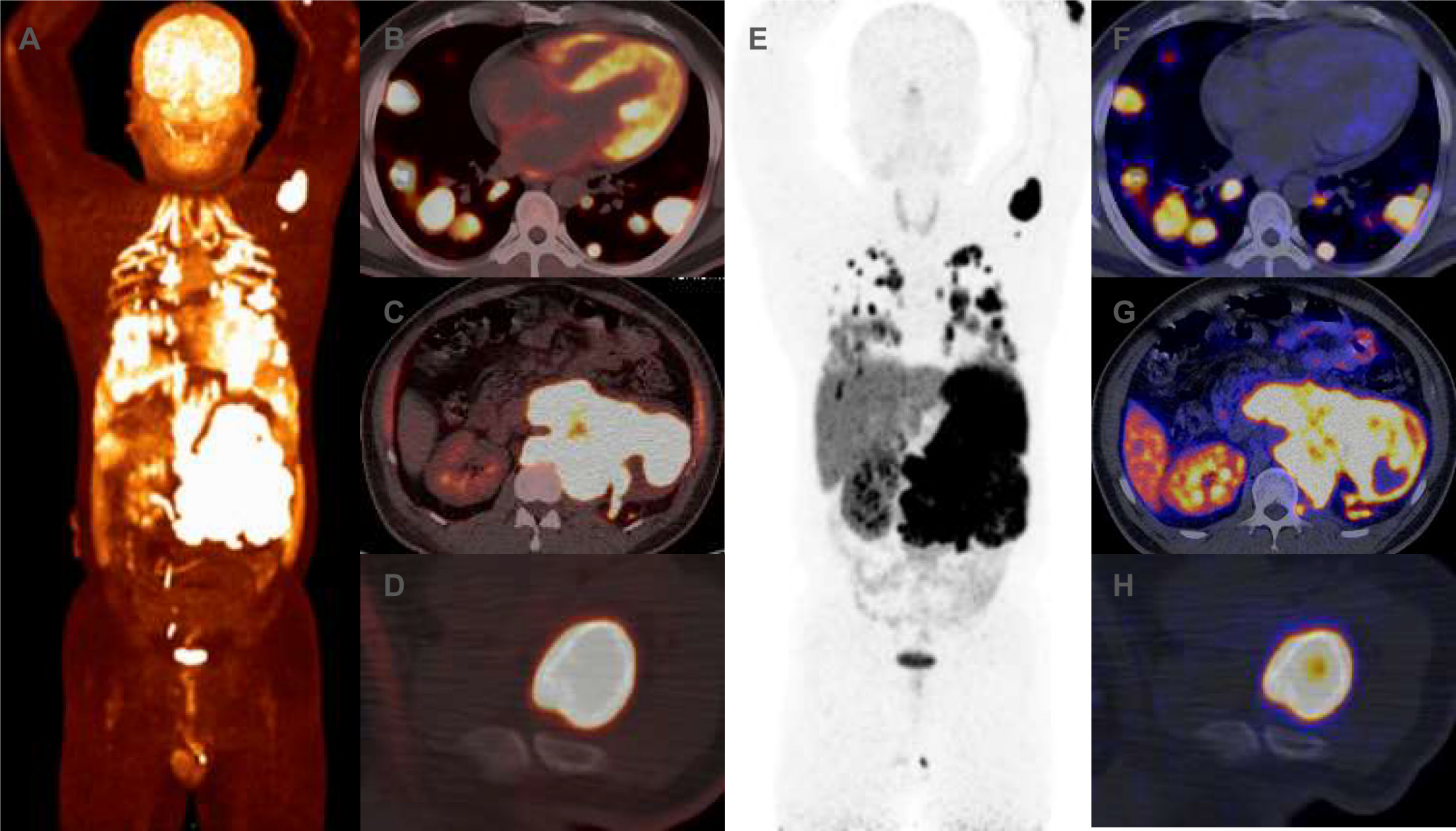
(A–F) Prior to CVD chemotherapy, 18F-FDG positron emission tomography (PET-CT) revealed increased uptake of 18F-FDG in the left retroperitoneal mass, lung masses and humeral head. The maximum cross-sectional area of the left retroperitoneal mass was approximately 166 × 99mm, with maximum SUV sizes of 21.49. (G–L) Prior to CVD chemotherapy, 68Ga-DOTATATE PET-CT revealed increase uptake of 68Ga-DOTATATE in the left retroperitoneal mass, lung masses and humeral head. The maximum SUV sizes of the left retroperitoneal mass was 36. 94.
